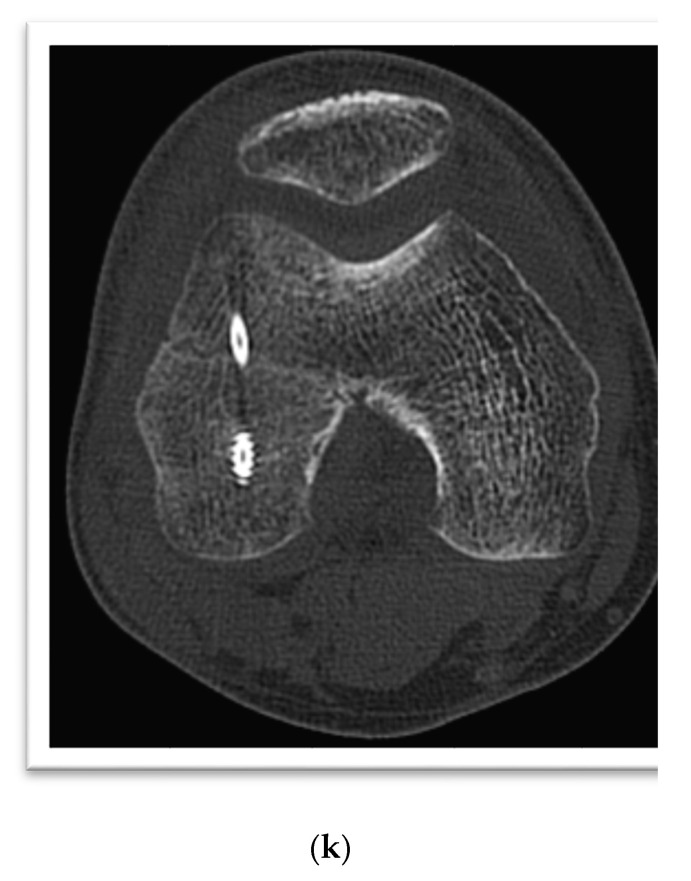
(a-k): (a) AP and (b) lateral radiograph of a Hoffa type fracture of the lateral femoral condyle, (c) sagittal and (d) axial assessment of the fracture morphology based on computed tomography (CT), (e) evaluation and (f) reduction for (g) anatomic reconstruction enables (h) arthroscopic assisted internal fixation; (i) AP and (j) lateral radiographic validation of screw positions and (k) follow-up CT.
